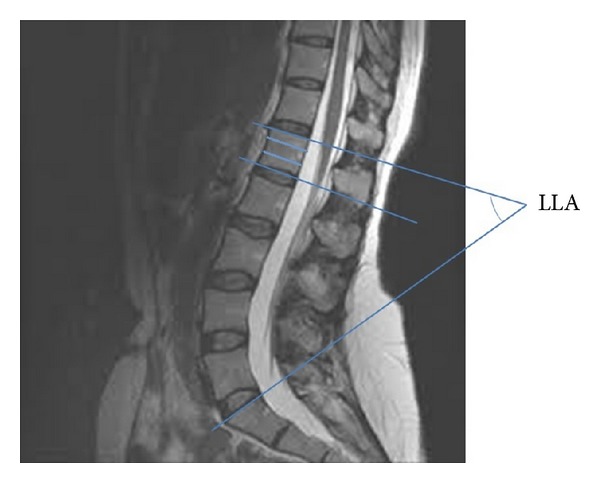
T2 weighted MRI of the lumbar spine showing the method of determination of the CMLT in relation to a vertebral segment.
